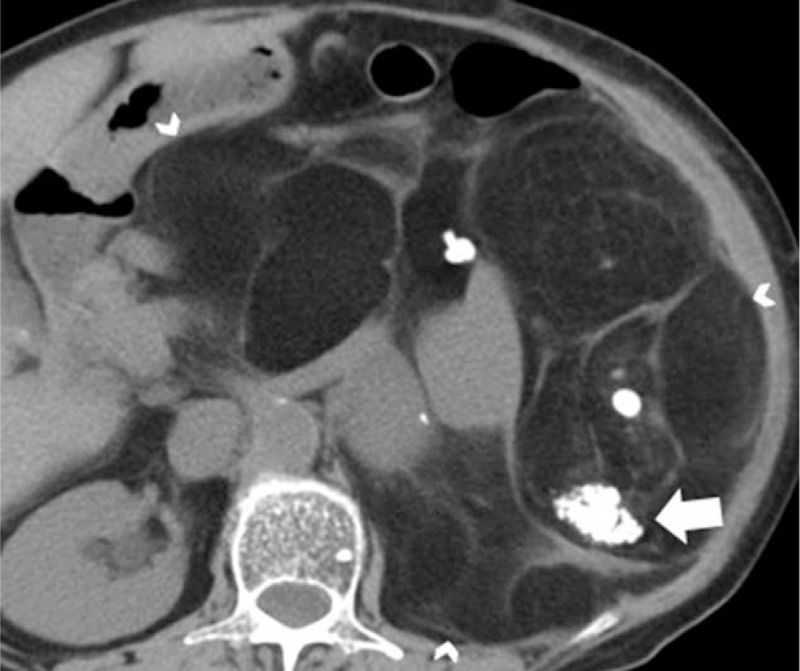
A 67-year-old woman with a large left retroperitoneal liposarcoma. Non–contrast-enhanced computed tomography scan shows a large fat-containing mass (white arrowheads) with coarse calcification (white arrow).
